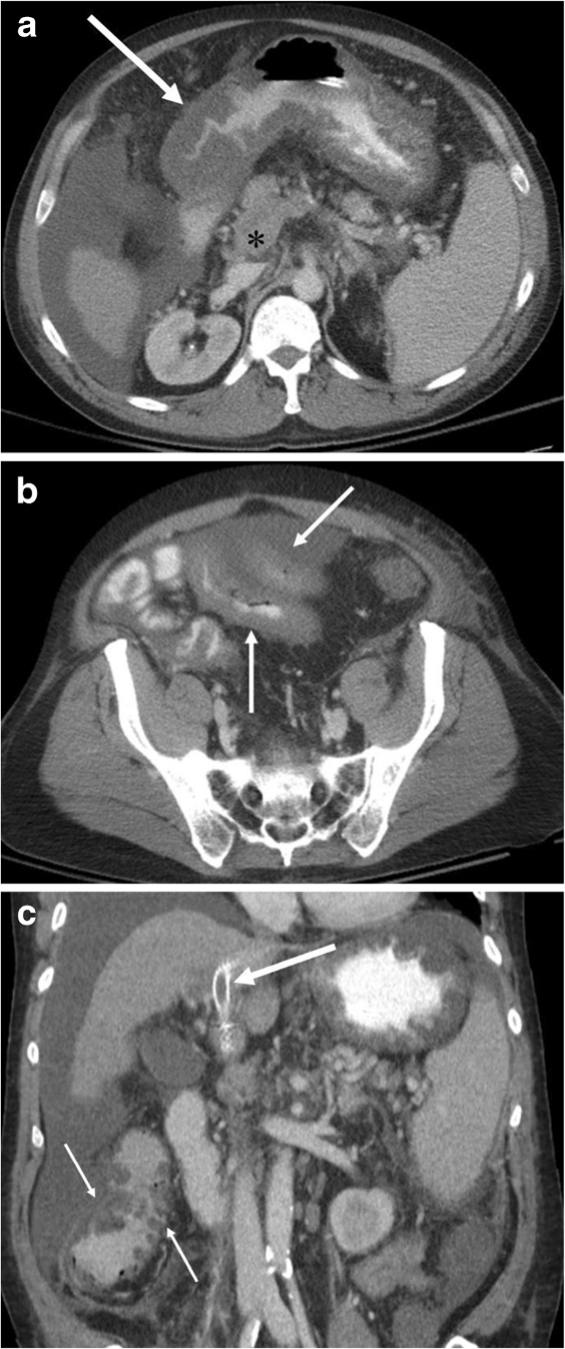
A 55-year-old male with liver cirrhosis presenting with portal venous thrombus and resultant small bowel ischaemia and congestion of the gastrointestinal tracts. a The venous phase of the axial CE-CT shows marked oedematous wall thickening of the gastric antrum (*arrow*). The splenoportal confluence (*asterisk*) is poorly opacified. Ascites and splenomegaly are also noted. b The axial CE-CT at the level of the pelvis shows wall thickening of the small bowel (*arrows*). Laparotomy found necrosis of the small bowel. c The coronal reformatted image shows an occluded transjugular intrahepatic portosystemic shunt (TIPS) stent (*large arrow*). The portal vein is not opacified at the level of the hepatic hilum due to thrombus. The *ascending colon* showed oedematous wall thickening (*small arrows*)
